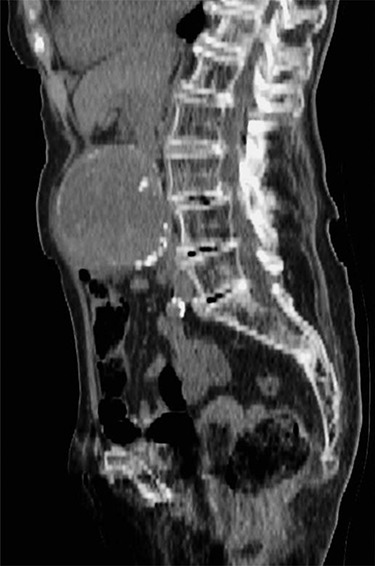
CT Aortogram demonstrating 8-cm infrarenal aneurysm.
